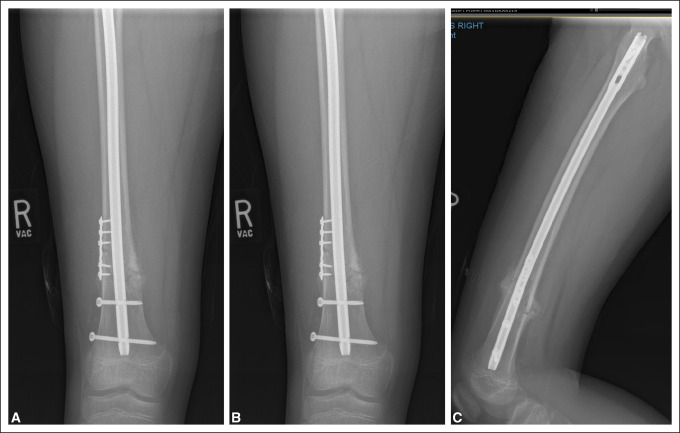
Radiographs obtained 6 weeks postoperatively demonstrating healed femoral shaft fracture with rigid intramedullary nail fixation.
